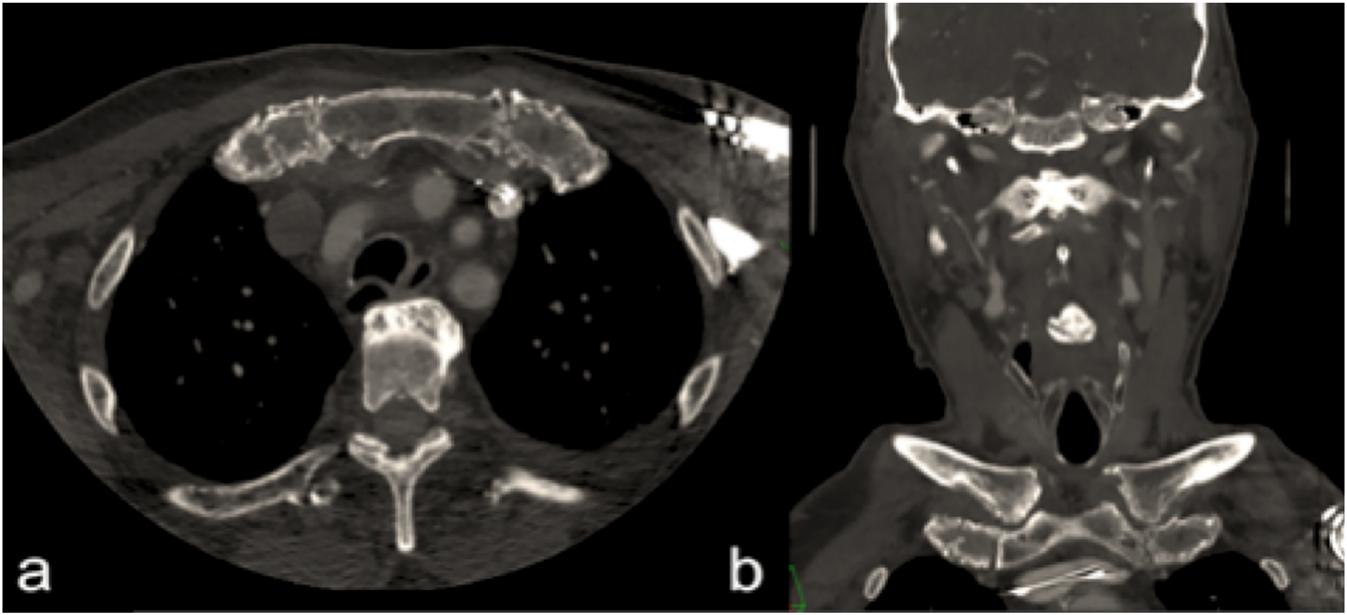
Typical case of Tietze syndrome (CT images, bone view): young man patient 30 years old, with costal pain and no history of inflammatory condition, autoimmune or neoplastic condition. Tietze syndrome is a benign condition characterized by an inflammation of the costosternal cartilage, often with hypertrophy. a Axial CT image (bone window) shows focal swelling and sclerosis of the second costosternal joint, with surrounding soft tissue thickening. b Coronal reformatted CT image confirms enlargement and sclerosis of the costal cartilage at the same level, consistent with Tietze syndrome
